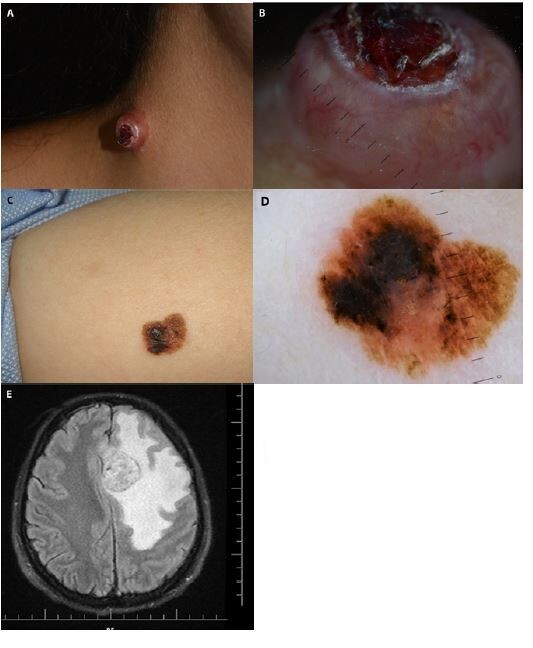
Case reports. (A) Case 1: 18-year-old woman diagnosed with pathology confirmed nodular melanoma with a Breslow thickness of 7.8 mm 6 months after ablative laser, and intralesional steroid injections. (B) Case 1: Dermoscopy showing a nodular mass with central hemorrhagic ulcer, serpentine-branched vessels on a pink structureless area, and remnants of brown pigmentation. (C) Case 3: Clinical image of a superficial spreading melanoma with a Breslow thickness of 0.75mm on the leg of a 33-year-old female. The lesion was first treated with chemical peels and reappeared after 3 months expanding continuously. (D) Case 3: Dermoscopic image showing a well demarcated lesion with chaos of border abruptness, eccentric gray-black structureless area, and white lines. (E) Case 4: Magnetic resonance imaging showing a melanoma metastasis in the brain causing severe edema and midline shift. The patient recalled non-surgical removal of a pigmented nevus from his leg
